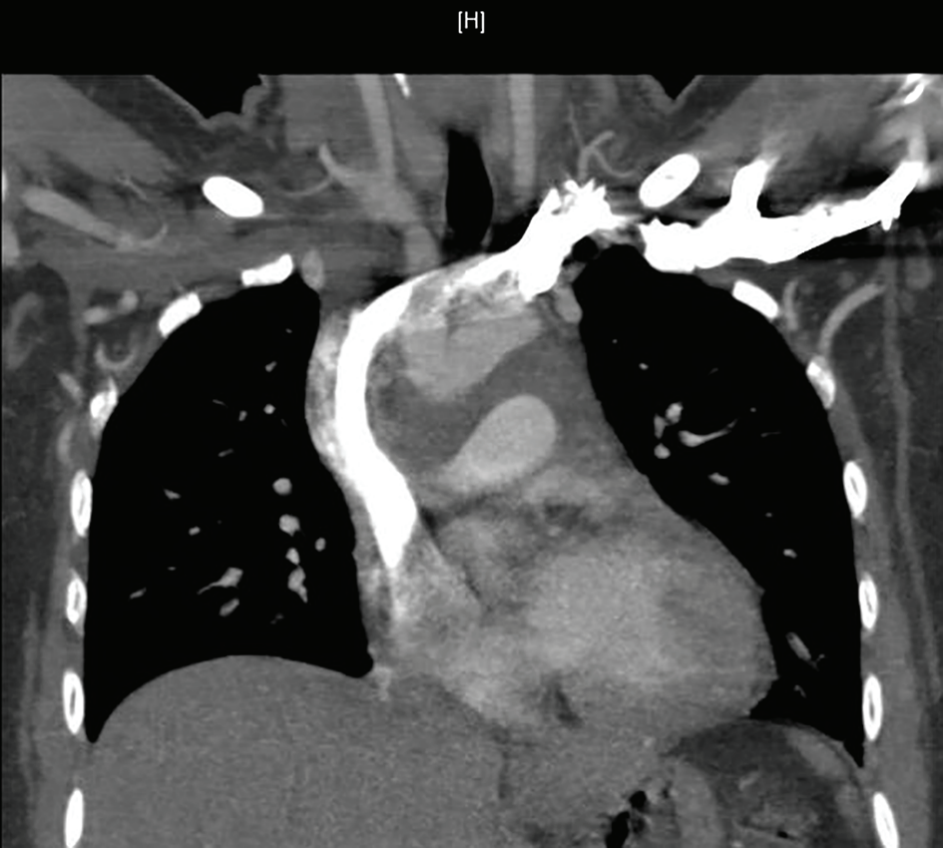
Computed tomography (CT) of chest showing mediastinal mass and mediastinal lymphadenopathy.
